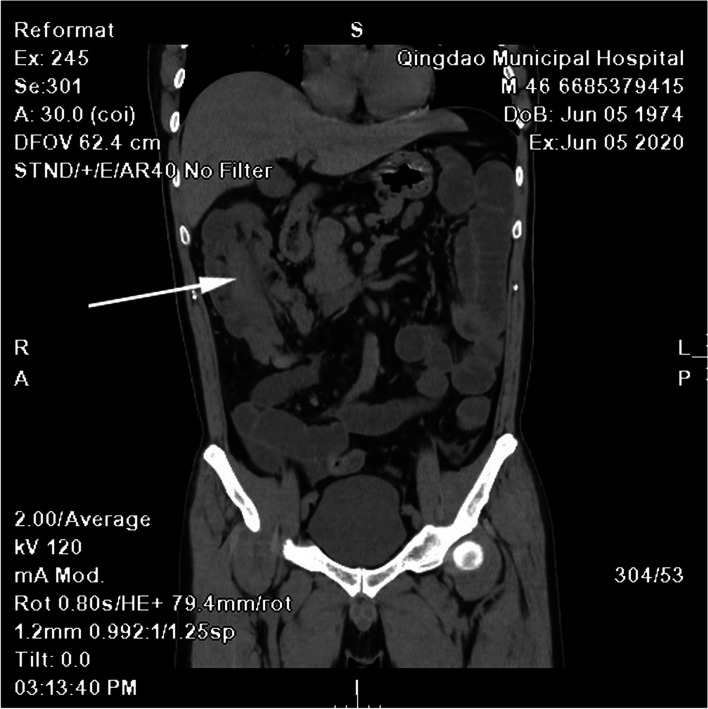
Abdominal computed tomography view indicated ascending colon intussusception (grey arrow)
